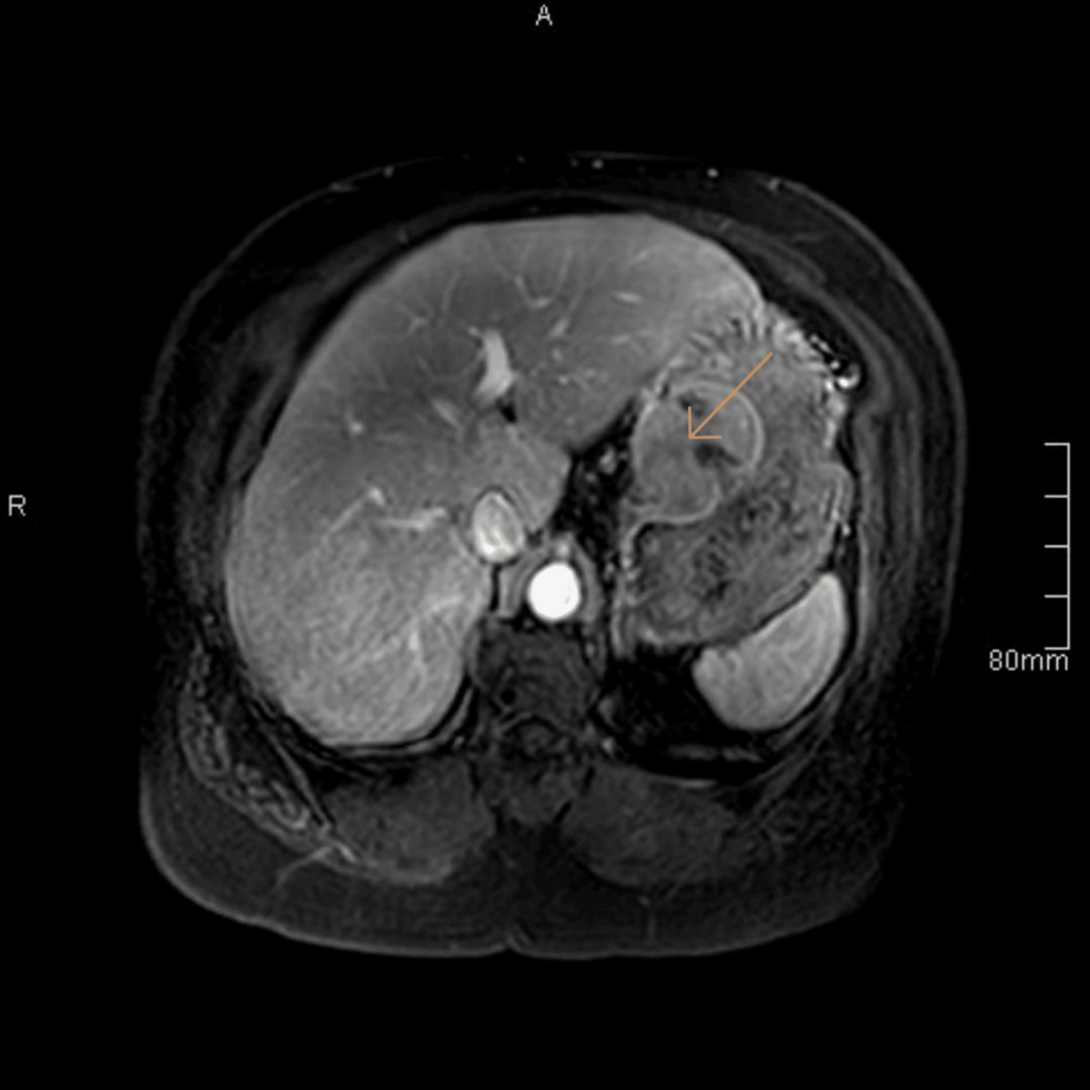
Contrast-enhanced T1-weighted MRI (post-contrast images) demonstrates heterogeneous enhancement and shows no definite invasion or extra gastric extension
